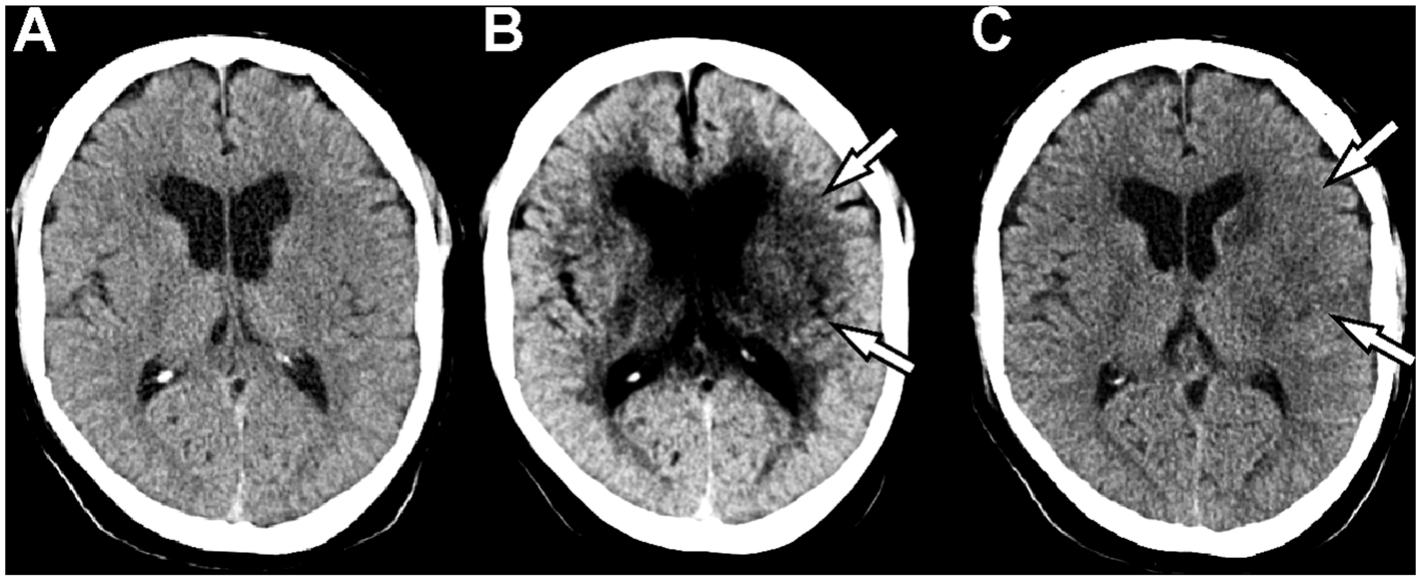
50-year-old male patient with ischemic stroke of the left hemisphere due to occlusion of the left internal carotid artery (ICA). The NECT (A) does not show a significant infarct demarcation, whilst the “best contrast” optimized images (B) show a demarcation of the left basal ganglia and the insular cortex (arrows), which was confirmed by follow-up CT (C).
